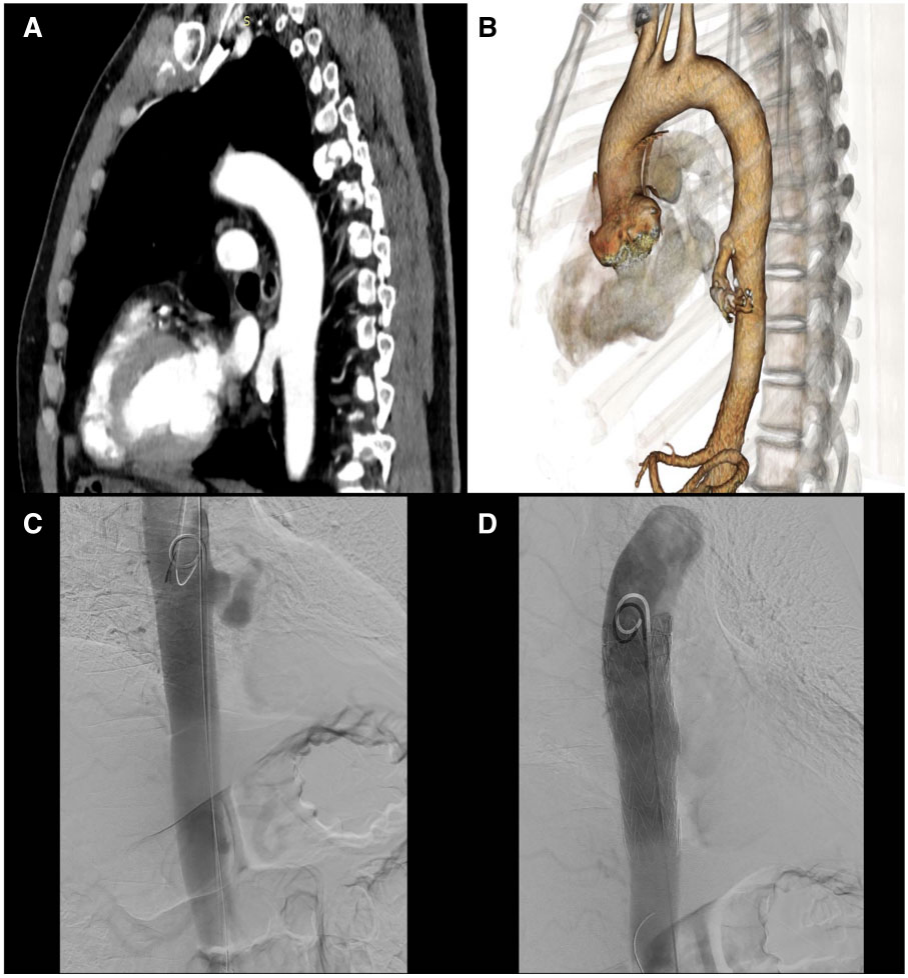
(A) Computed tomography scan of an aberrant aneurysm artery; (B) 3-dimensional computed tomography scan of a vascular reconstruction; (C) aberrant vessel as seen on an angiography examination; and (D) endograft angiographic check.
